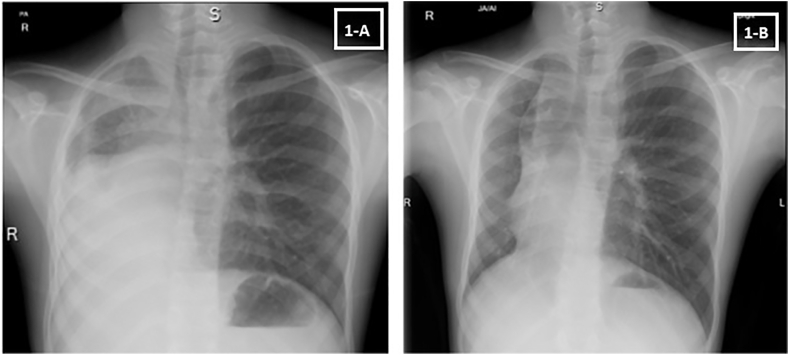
(A) Chest X-ray at presentation (B) Chest X-ray after ablation.
